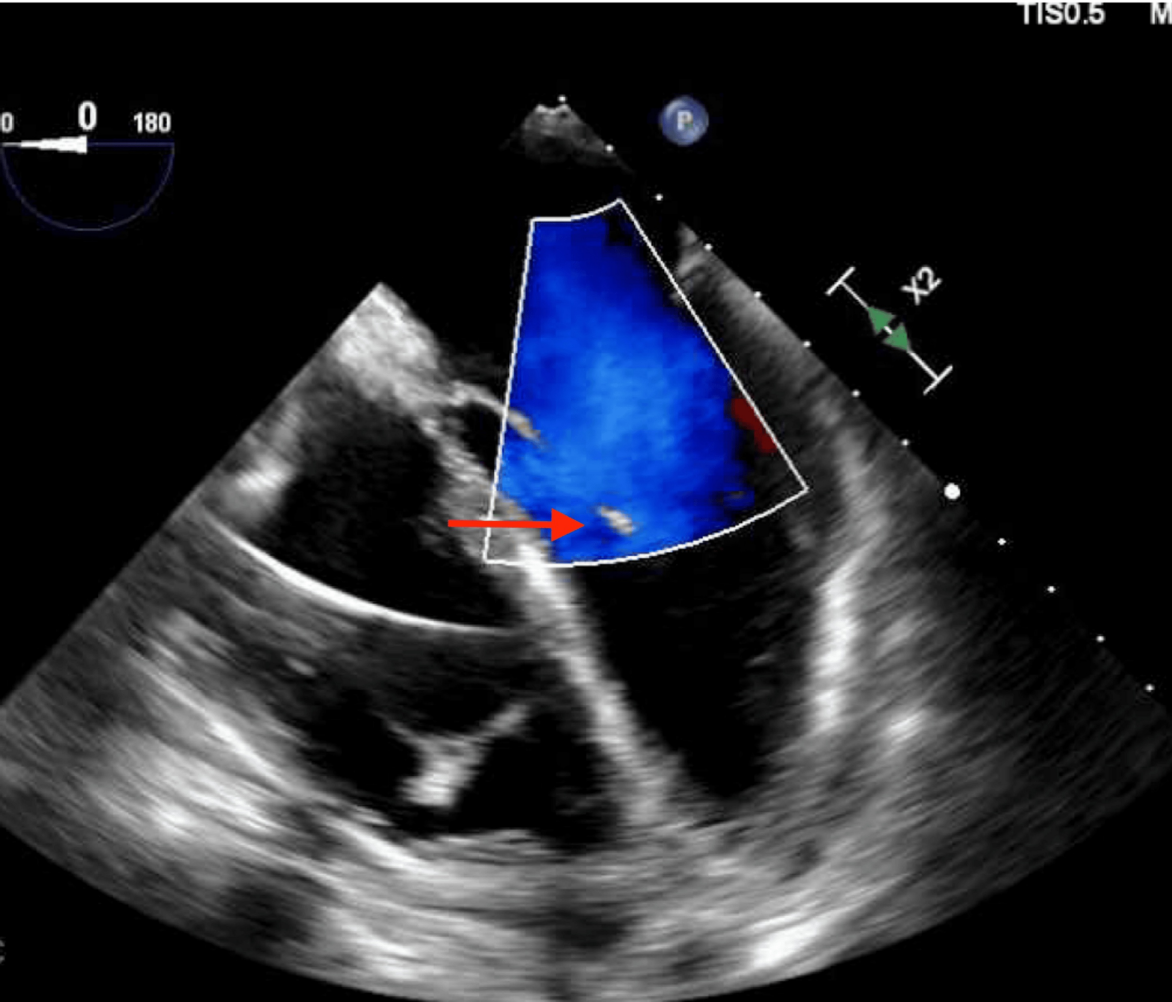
Echocardiogram The RV was normal in size, with a basal diameter of 4.0 cm (normal <4.2 cm) and preserved systolic function. A pacing lead was visualized in the RV. A very small mobile density, measuring approximately 2 mm, was seen attached to the RV endocardium adjacent to the lead. This finding is more likely a fragment of RV myocardium than a vegetation. RV, right ventricle
